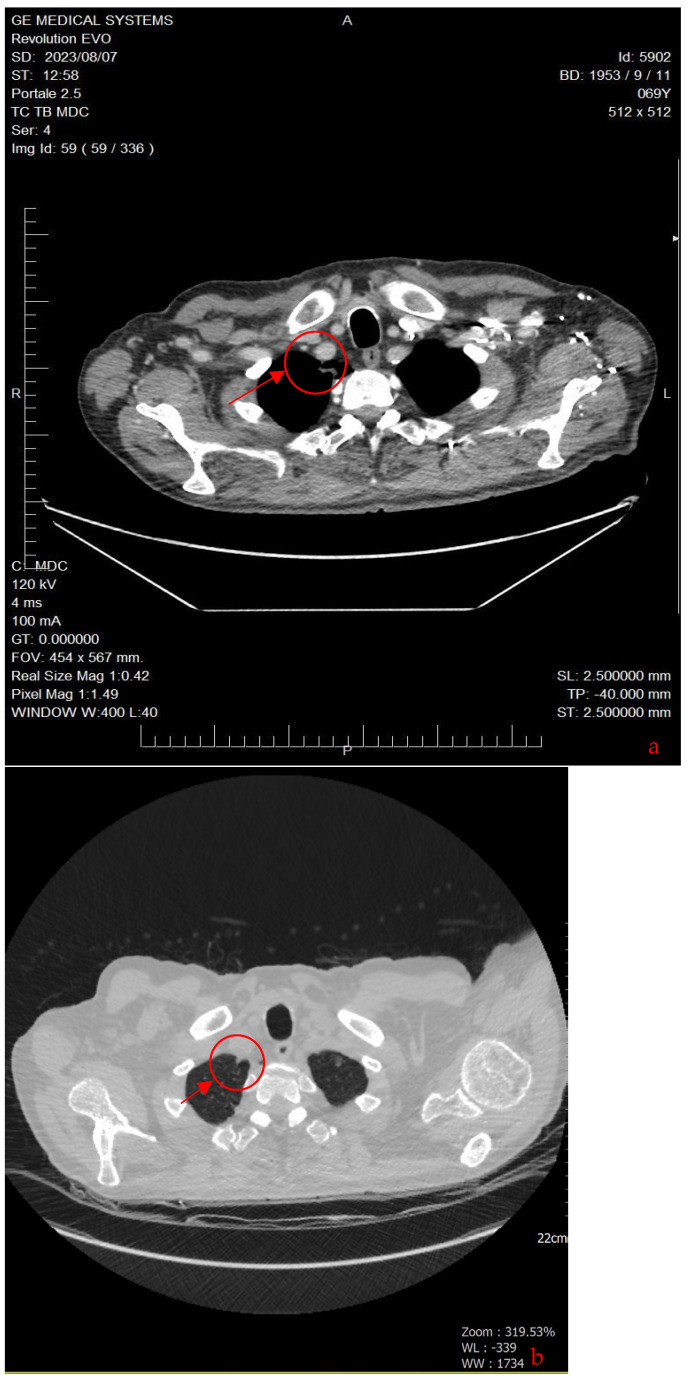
The axial CT scan shows that the solid, nodular-type, expansive process in the right upper lobe of the lung is no longer visible (red circle). This means that the nodule has disappeared or shrunk to a size that is no longer detectable on the CT scan (a). To the right: lung windowing (b) (2023).
